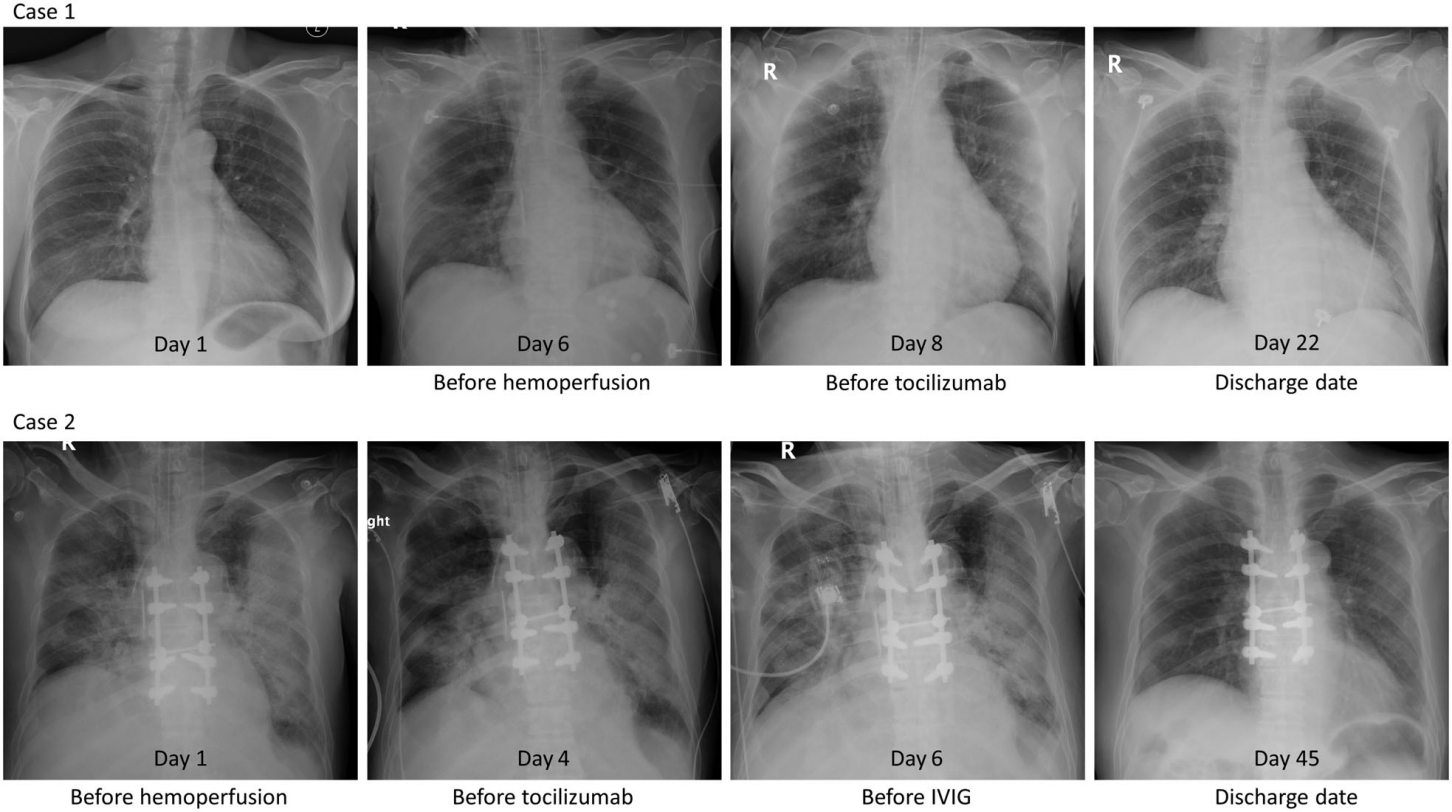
Chest radiographs on initial presentation, on the day before each adjunctive therapy, and on discharge date.
